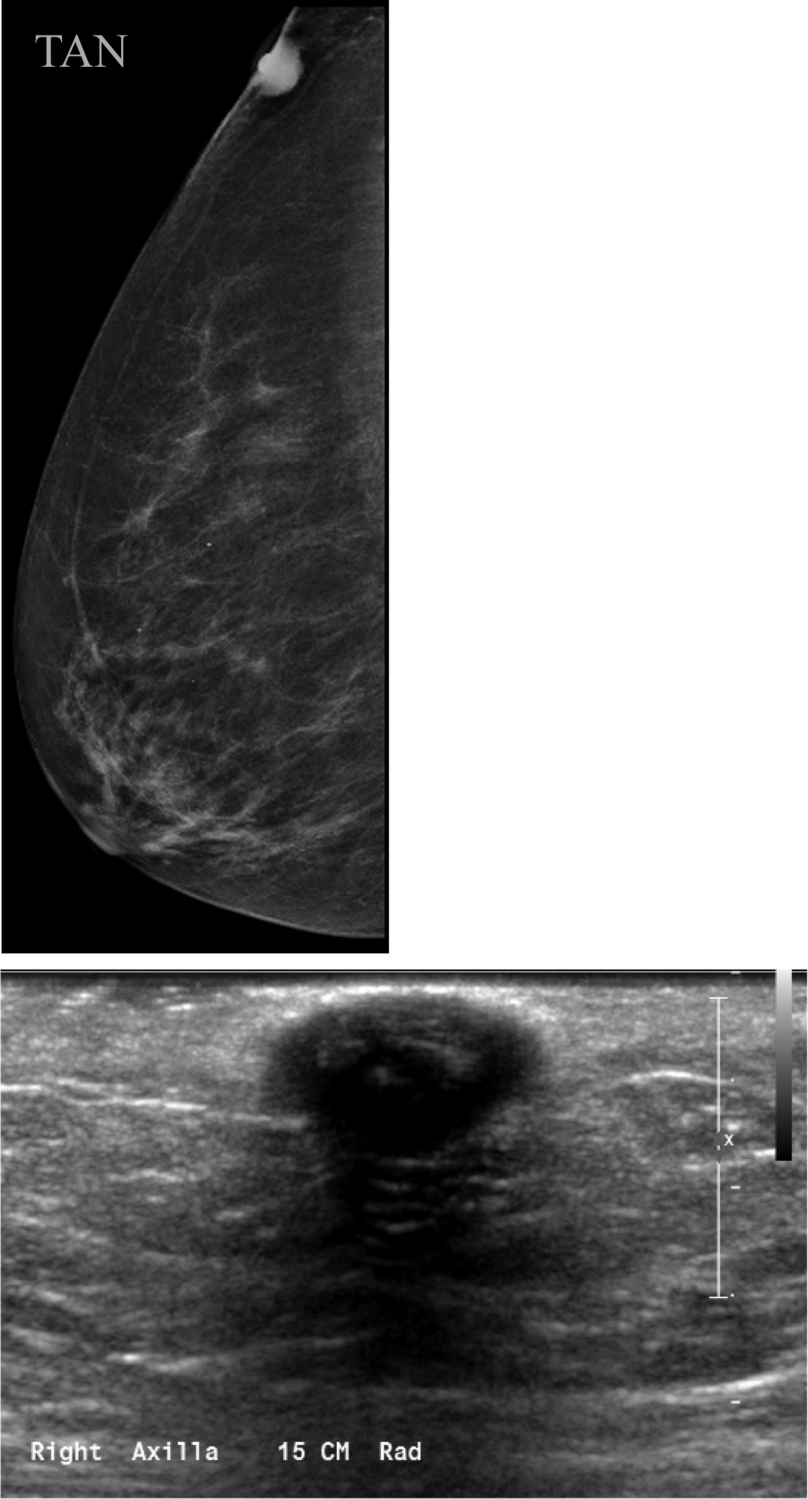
Sebaceous cyst or epidermal inclusion cyst. a Tangential view reveals that this lesion is located within in the skin. b Ultrasound confirms a hypoechoic mass within the skin
